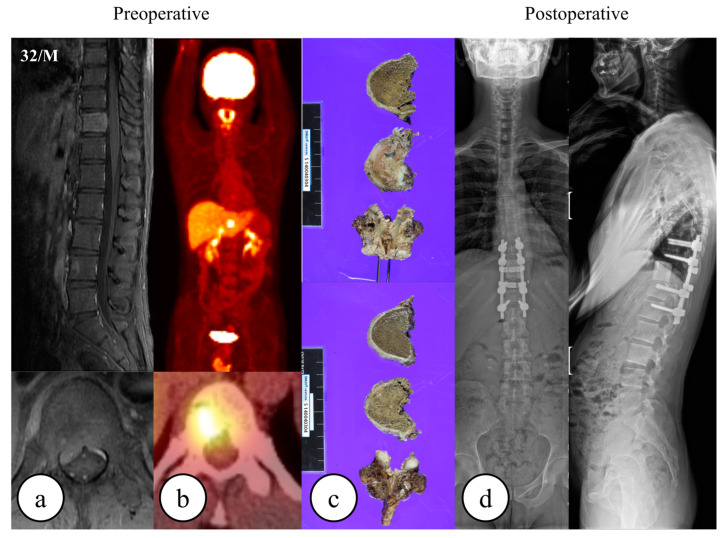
A 32-year-old male underwent total en bloc spondylectomy for a solitary metastasis of adrenal cortical carcinoma at T11. The following images are provided: (a) preoperative MRI, (b) preoperative whole-body FDG-PET CT, (c) gross pathology, and (d) postoperative radiography.
